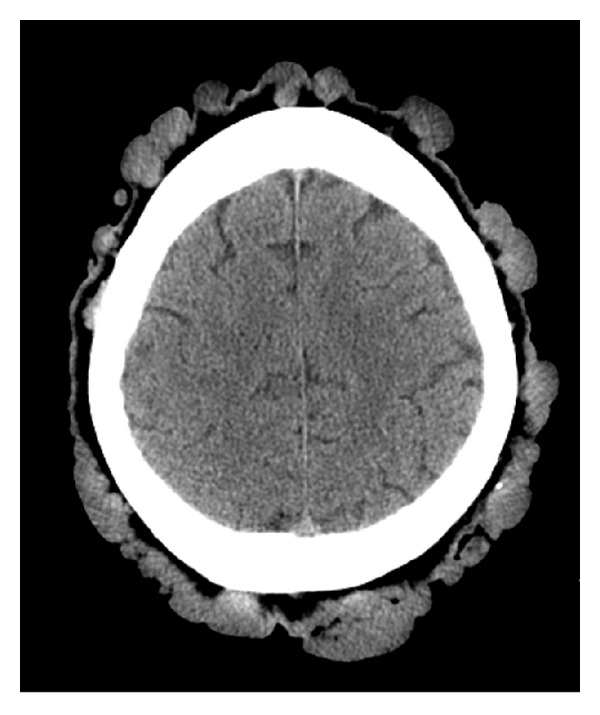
Noncontrast head CT: multiple nodular smooth surfaced well-demarcated soft tissue lesions arising from the scalp.
